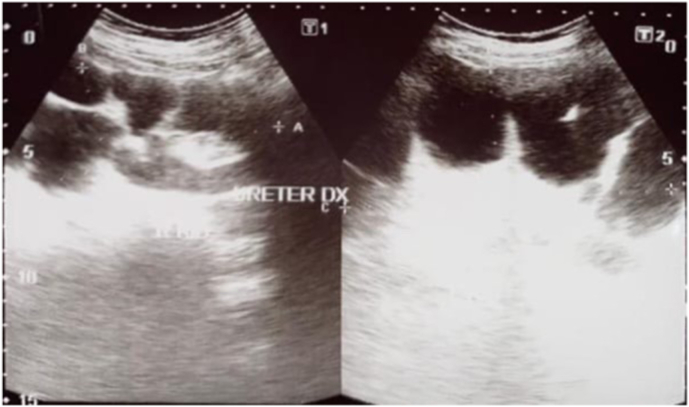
Ultrasonography examination showing hydronephrosis on both kidney.
